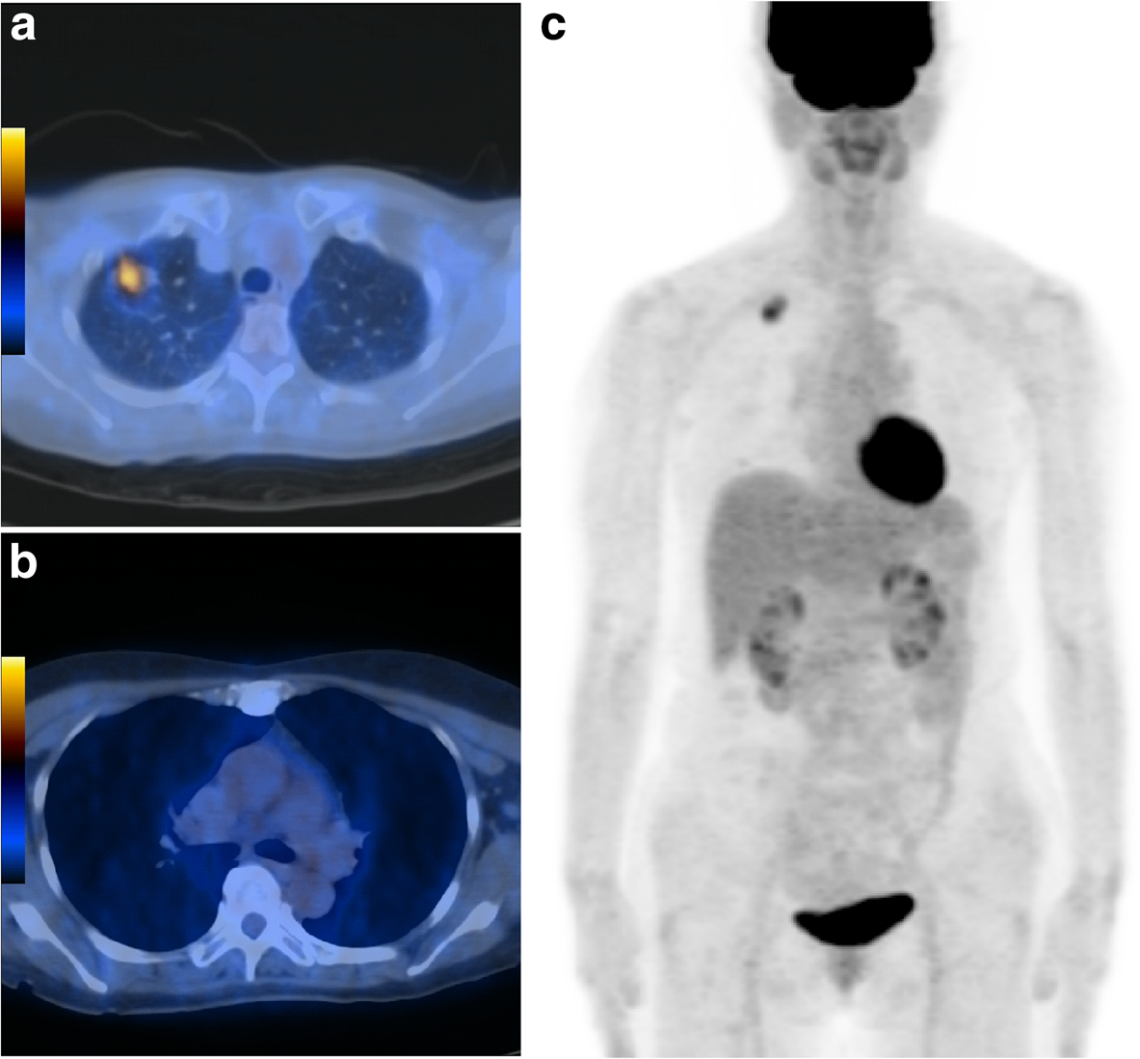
Images of a 60-year-old female patient with adenocarcinoma. The primary tumor size measured on chest CT was 2.4 cm. a Transaxial PET/CT fusion image shows a hypermetabolic pulmonary nodule in the right upper lobe. The SUVmax, MTV and TLG of the primary tumor were 4.3, 3.5 cm^3^, and 9.9, respectively. b and c Transaxial PET/CT fusion image and maximum-intensity-projection image showing no significant FDG uptake suggestive of metastasis; however, right hilar lymph node metastasis was confirmed after surgery
